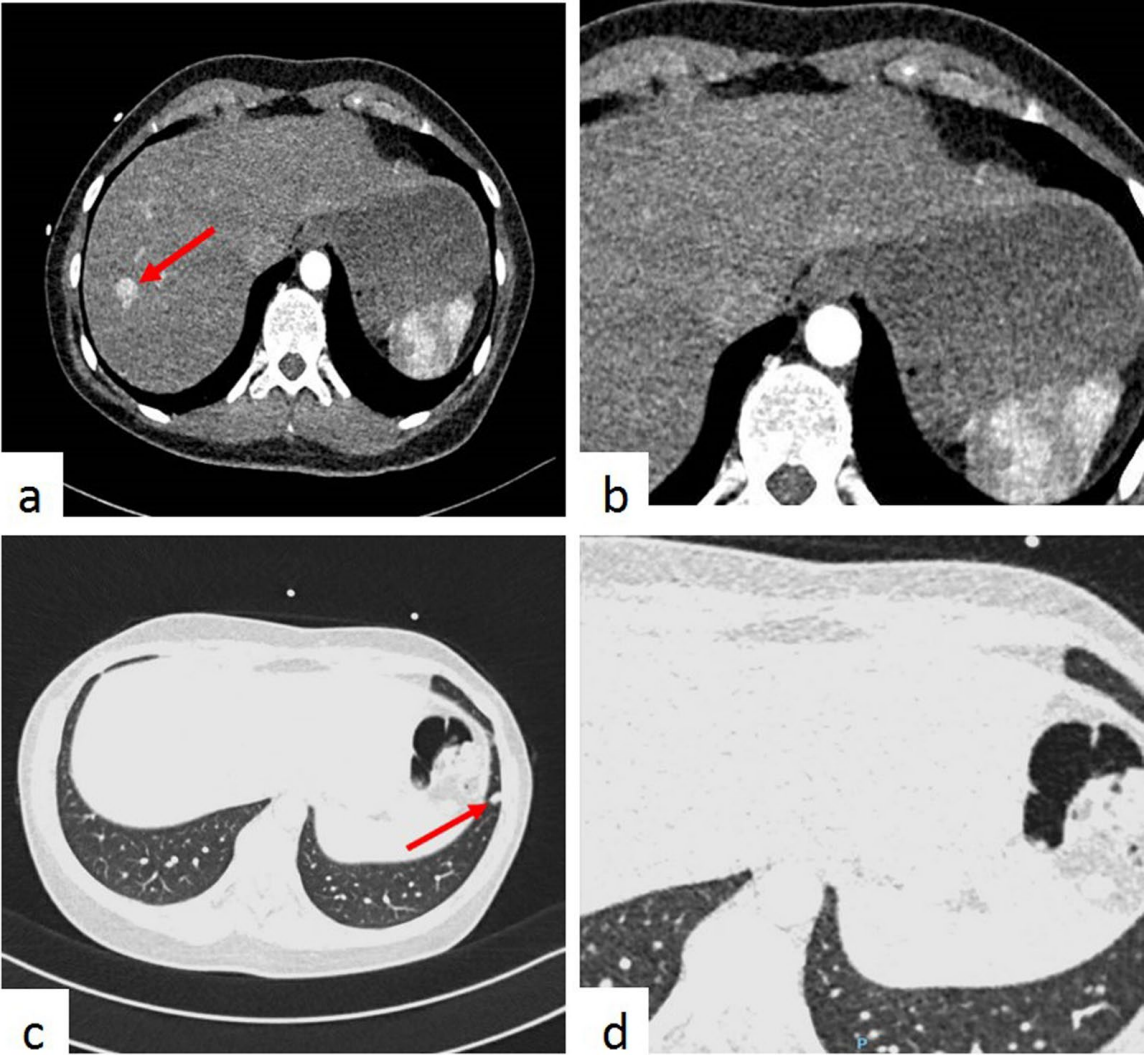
Examples of a moderate liver and a moderate lung IF in different field of views. a An example of a moderate liver IF (a hypervascular lesion) in a full field of view (FOV) with a ‘soft tissue’ window level (C:40 W:400). b The same CTA as a, but in a small FOV, in which the moderate liver IF falls beyond the area of this reconstruction. c An example of a moderate lung IF (a lung nodule) in a full FOV with a ‘lung’ window level (C:-600 W:1200). d The same CTA as c, but in the small FOV, in which the lung nodule falls beyond the area if this reconstruction
